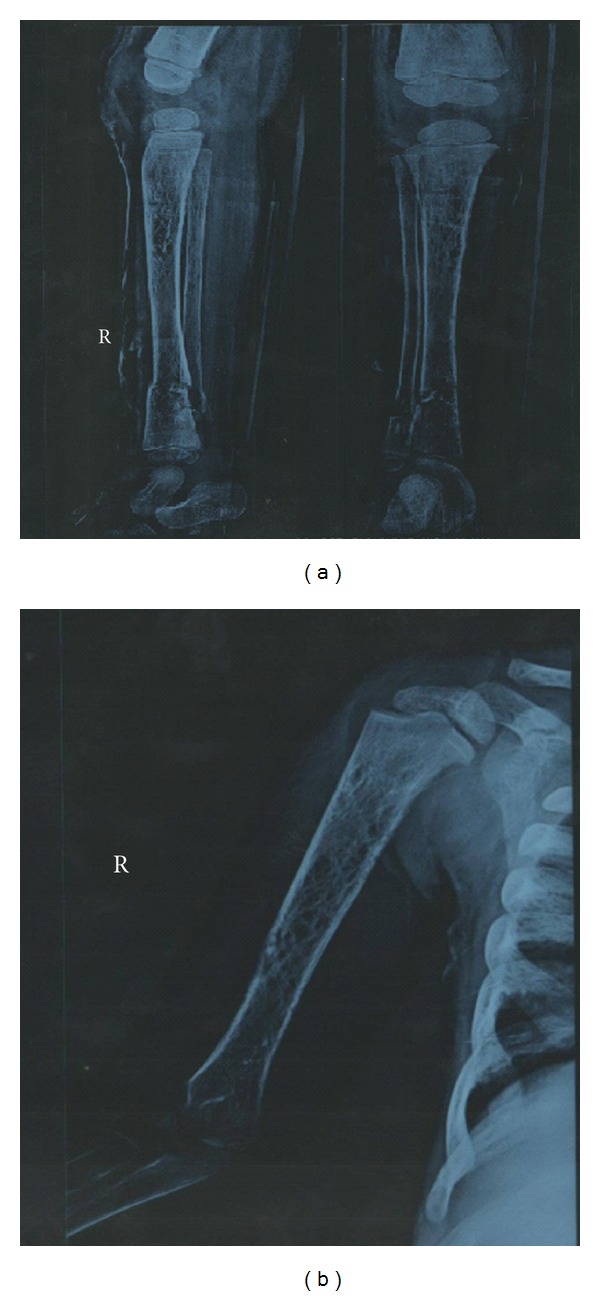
(a) X-ray of RT T/F AP/LAT shows osteoporosis and fractures. (b) X-ray of RT humerus shows severe osteoporosis.
